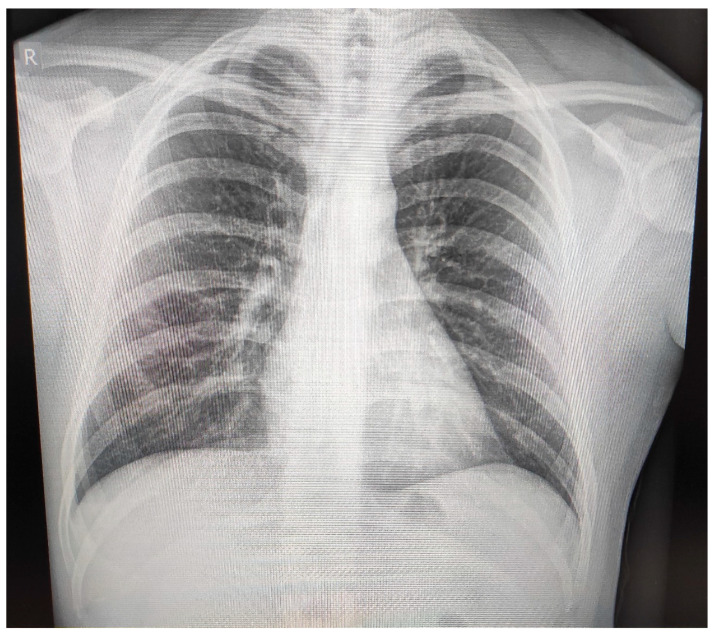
Chest radiograph of the patient. R, right.
